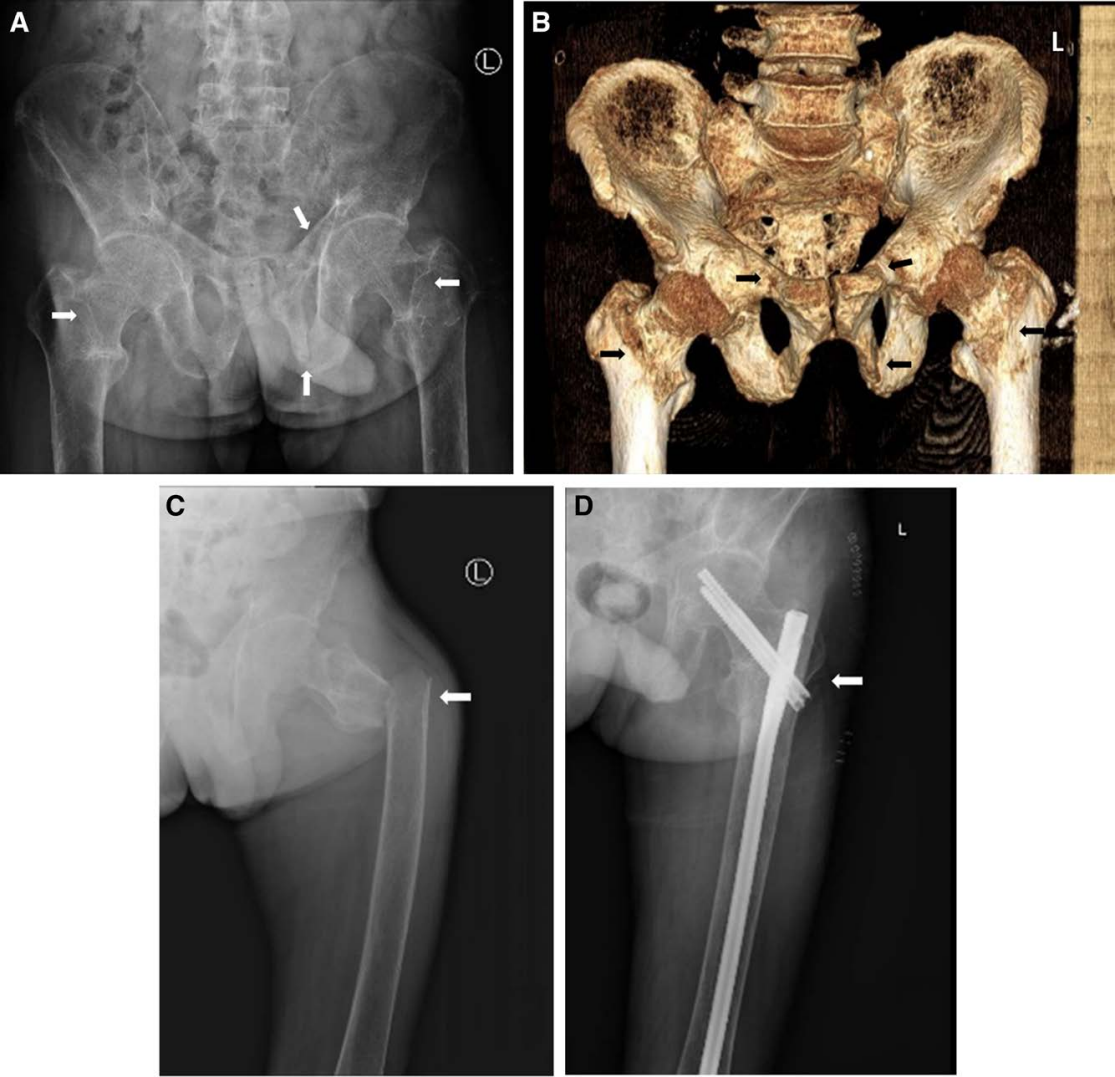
Pelvis radiograph (A) revealed pseudofractures of the bilateral femoral necks, irregular bone substance of the left superior and inferior ramus of pubis, and osteomalacia. computed tomography 3-dimensional imaging of the pelvis (B) showed bone discontinuity of the right superior ramus of pubis and left superior and inferior ramus of pubis, osteoporosis, nonhomogeneous density of the pelvis, and suspected fracture of the bilateral femoral necks. Fracture of the left proximal femur (C, left hip radiograph) occurring during hospitalization, with intramedullary fixation of fracture by open reduction (D, postoperative radiograph).
